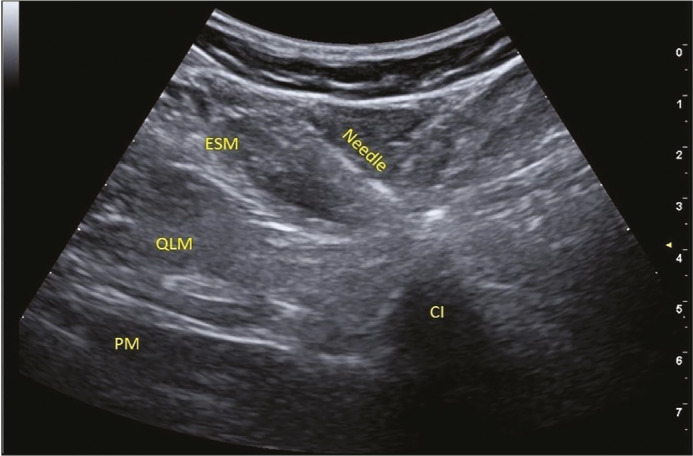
Quadro-iliac plane block-ultrasound view. CI, crista iliaca; ESM, erector spinae muscle; PM, psoas muscle; QLM, quadratus lumborum muscle.
